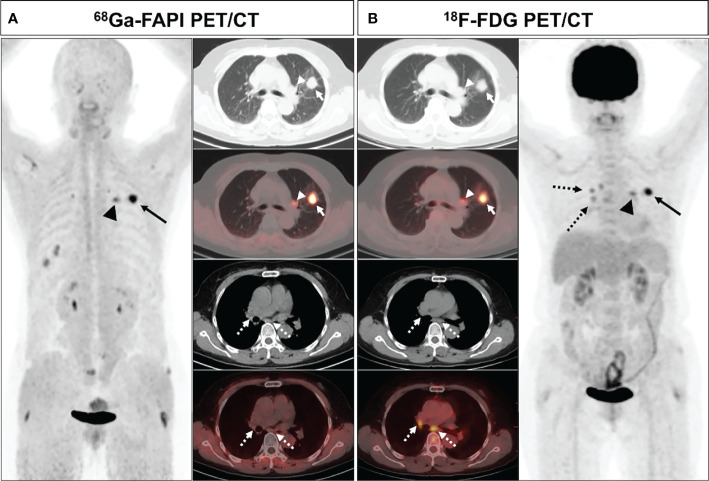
A 61-year-old female (patient 23) diagnosed with adenocarcinoma. ^68^Ga-FAPI PET/CT (A) revealed intense FAPI uptake in the primary tumor (solid arrows, SUVmax = 9.7) along with moderately increased uptake in the left pulmonary hilar lymph node (arrows, SUVmax = 5.0), whereas there was no FAPI uptake in the subcarinal and right pulmonary hilar lymph nodes (dashed arrows). ^18^F-FDG PET/CT (B) demonstrated intense FDG uptake in the primary tumor (solid arrows, SUVmax = 9.0) with moderate uptake in the left pulmonary hilar (arrows, SUVmax = 4.5), subcarinal, and right pulmonary hilar (dashed arrows, SUVmax = 3.5) lymph nodes. Pathological biopsy confirmed metastasis in the left hilar lymph node, while no metastasis was found in the subcardiac or right hilar lymph nodes.
